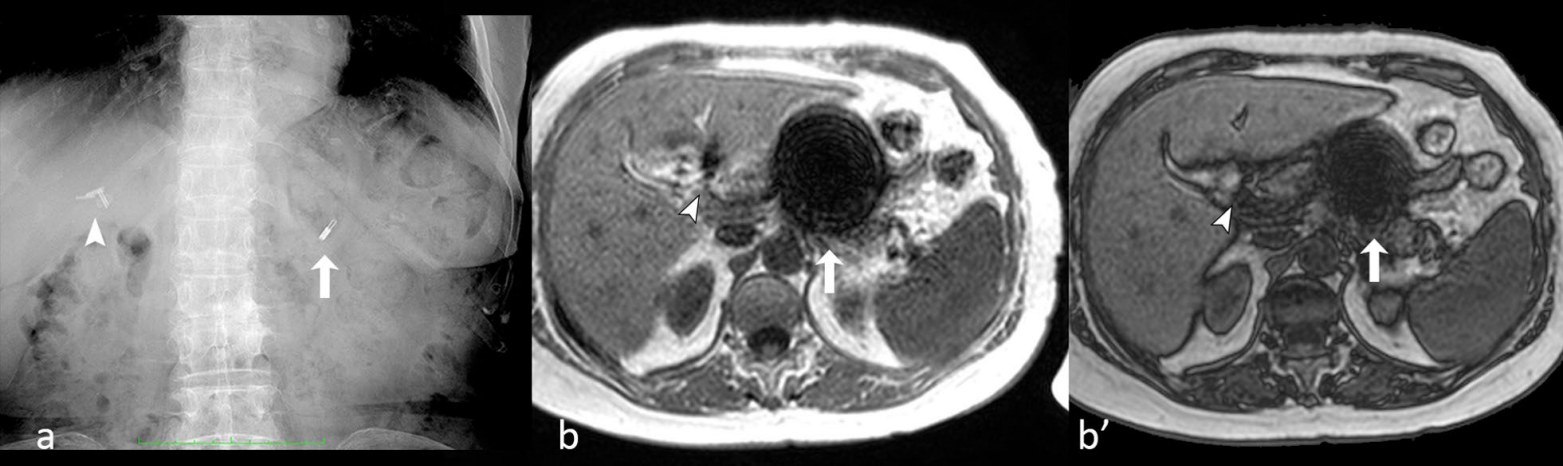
Abdominal X-ray (a) showing two metallic devices in the right (arrowhead) and left (arrow) upper quadrants, respectively. The devices cause magnetic susceptibility-related artifacts on in-phase (b) and opposed-phase (b′) gradient echo (GE) T1-w. images in the stomach (arrow) and hepatic hilum (arrowhead), respectively; the signal intensity loss is higher on in-phase image (b). It is worthy of note that the magnetic device in the stomach (a ferromagnetic steel clip) causes a much more prominent artifact than the device in hepatic hilum (a paramagnetic titanium clip by previous cholecystectomy)
